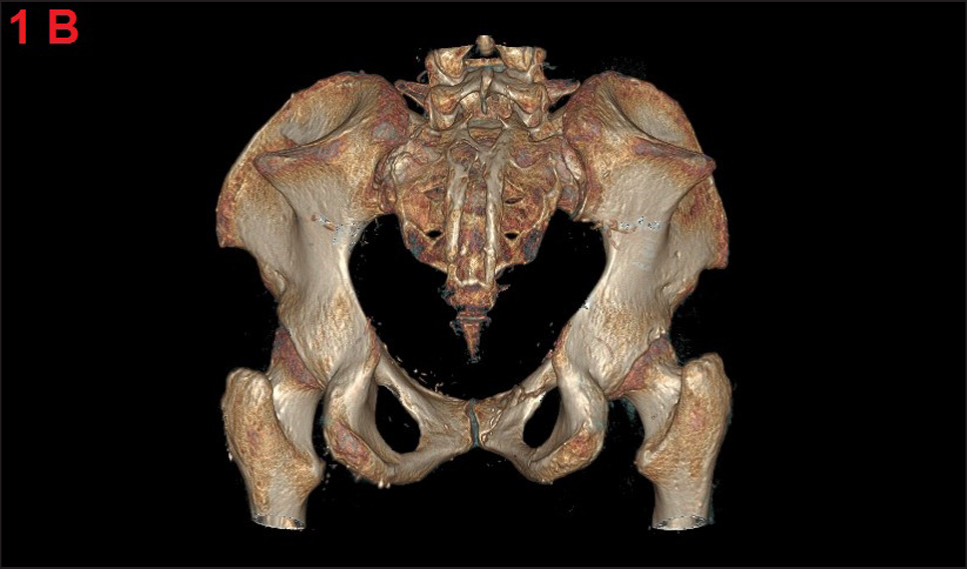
3D reconstruction image, posterior view, of the pelvic CT shows the bilateral iliac horns.
